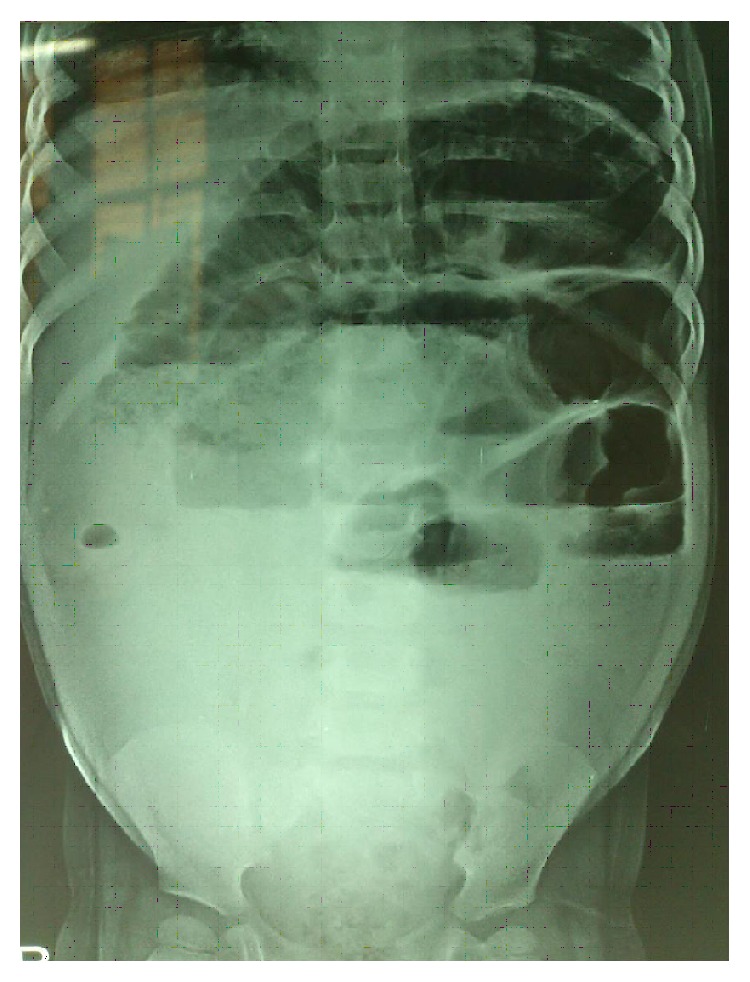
X-ray erect abdomen showing air fluid levels.
